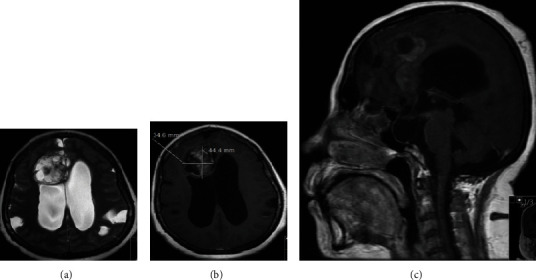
Axial view of a T2-weighted image with a right frontal intra-axial lesion measuring 34 × 44 mm in the transverse and anteroposterior dimensions, respectively. The lesion indents the ependymal surface of the right lateral ventricle. The lesion is heterogeneous with cystic loculations indicating necrosis; an associated bilateral ventriculomegaly of the lateral horns is appreciated (a). The lesion has a heterogeneous enhancement pattern in a T1-weighted image with contrast (b). T1 with contrast sagittal view revealing a scalloped corpus callosum with empty sella (c).
